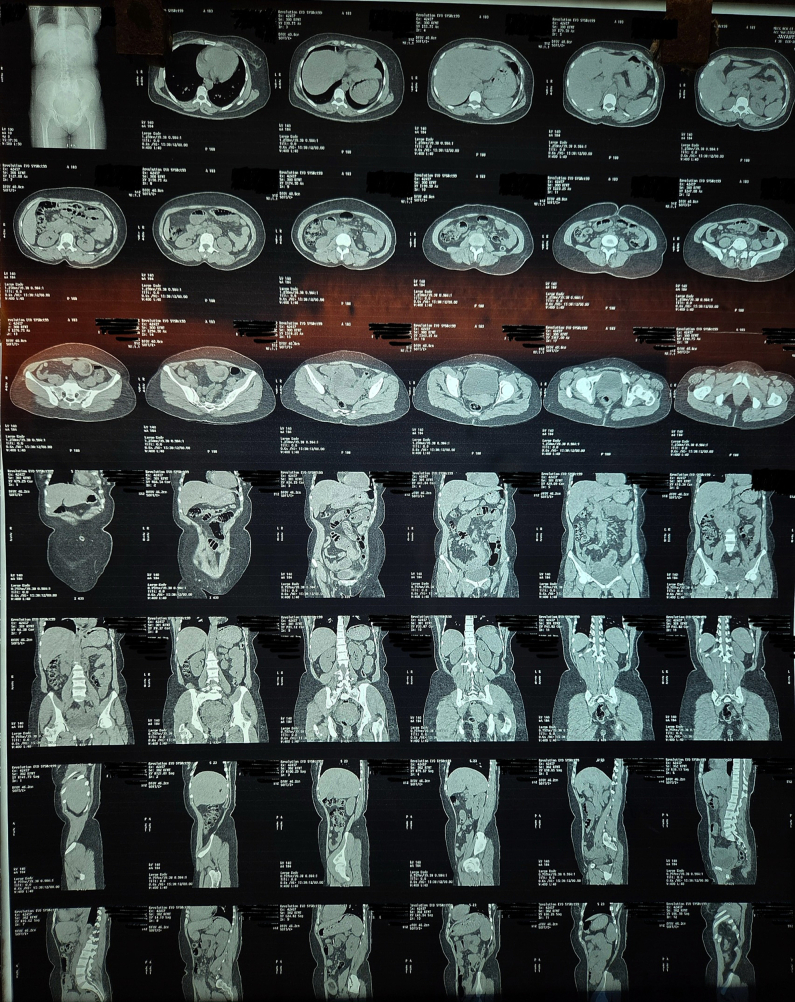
Non Contrast Computed Tomography of Abdomen and Pelvis (Zoomed Out).
